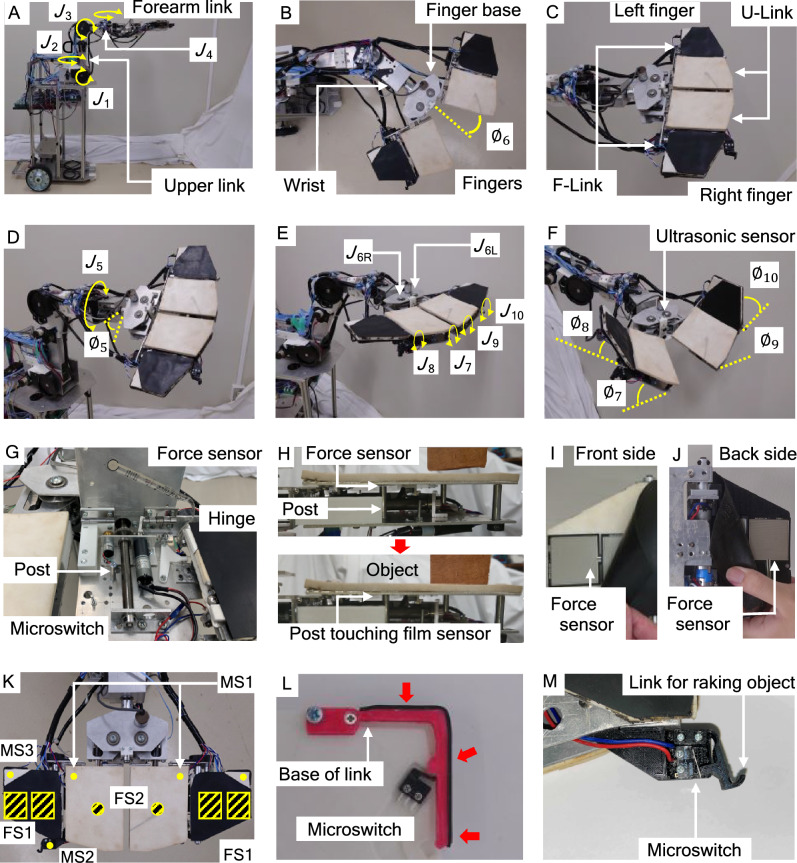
Mechanism and sensors of robotic hand. (A) Overview, (B) wrist and finger base ($${\mathrm{\varnothing }}_{6}$$: angle of finger), (C) forearm links (F-Links) and upper links (U-Links) of fingers, (D) angle in roll direction around *J*_5_ ($${\mathrm{\varnothing }}_{6}$$), (E) axes of finger base and fingers, (F) angles of finger axes ($${\mathrm{\varnothing }}_{7}-{\mathrm{\varnothing }}_{10}$$) and position of ultrasonic sensor on finger base, (G) force sensors inside U-Link, (H) action of U-Link for touching object, (I) force sensor on front side between two sponge rubber parts, (J) force sensor on back side between two sponge rubber parts, (K) arrangement of sensors (FS1 and FS2 are force sensors and MS1-MS3 are microswitches), (L) touch sensor system for raking an object designed in 3D-CAD, and (M) touch sensor system designed in 3D-CAD.
